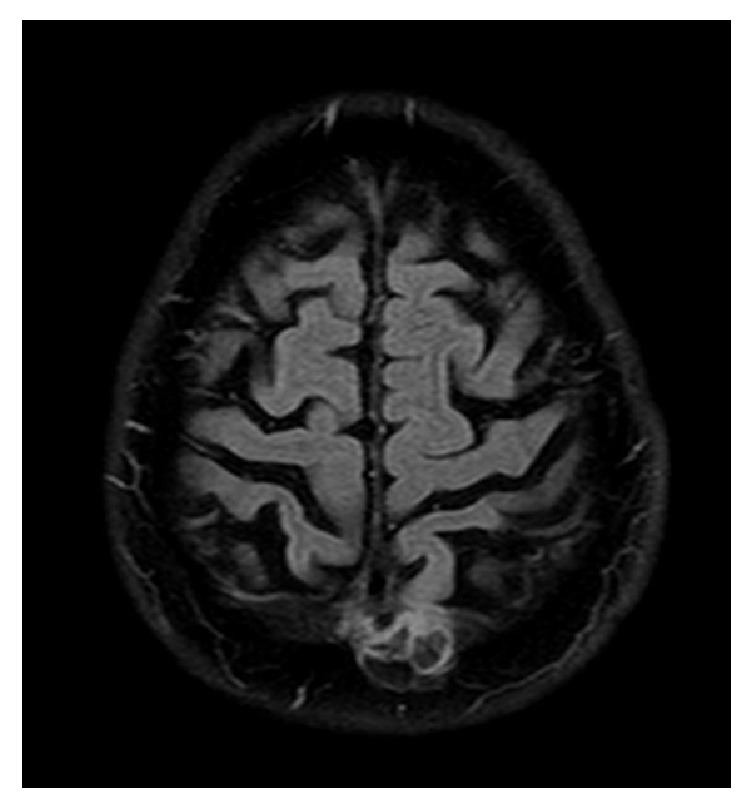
Axial T2-weighted FLAIR image shows hyperintensity of the brain herniation, probably due to prior infarction.
